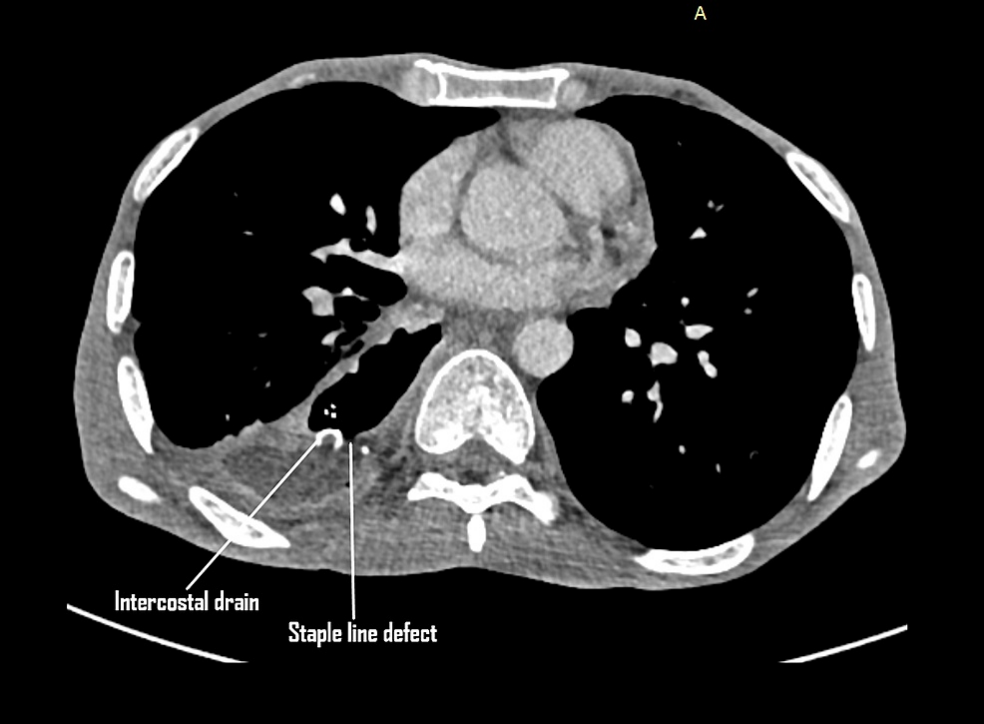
Axial cut from contrast-enhanced CT (CECT) thorax done on day nine showing an empyema in the right hemithorax. A defect in the conduit can be appreciated which is communicating with the empyema
